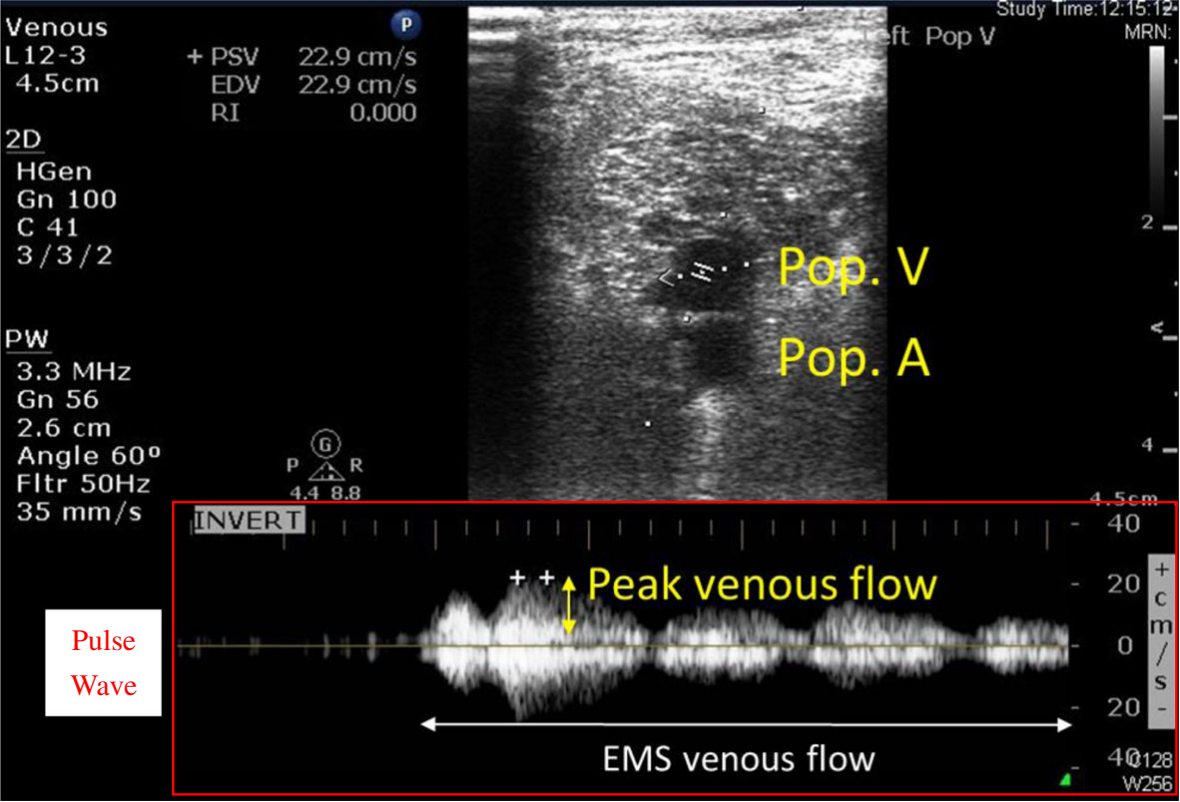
Representative screen shot from pulsed Doppler ultrasound for measurement of the peak venous velocity and diameter of the popliteal vein (Pop. V). Both were measured in the short-axis view. In this picture, the venous velocity was measured at the center of double line (denoted as *equal sign*) continuously and then the pulse wave was figured. The peak velocity was measured at the top of the pulse wave (denoted as *plus sign*)
